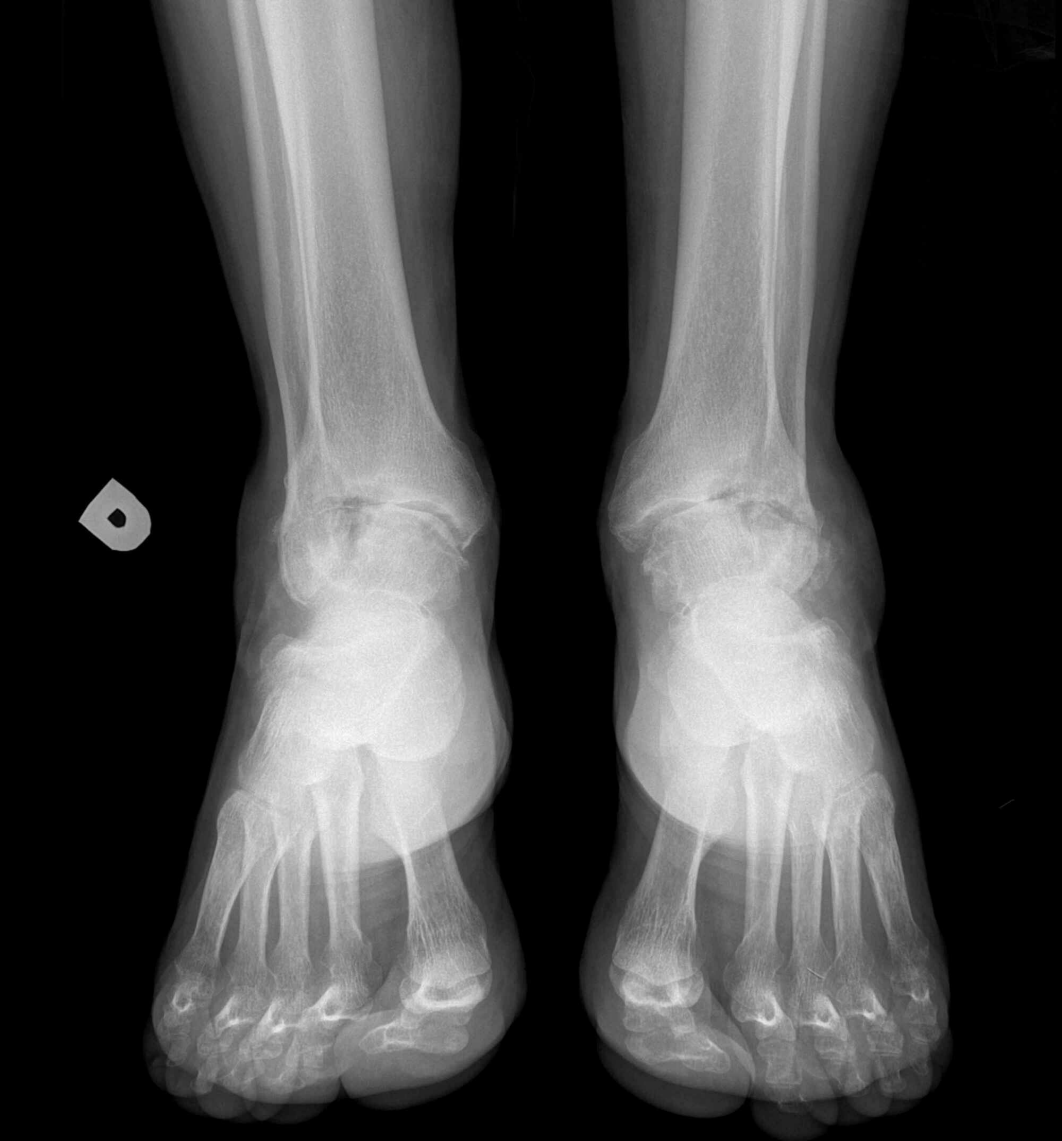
Preoperative radiographs Antero-posterior RX view of severe ankle degeneration, weight bearing RX was not possible due to patient pain
